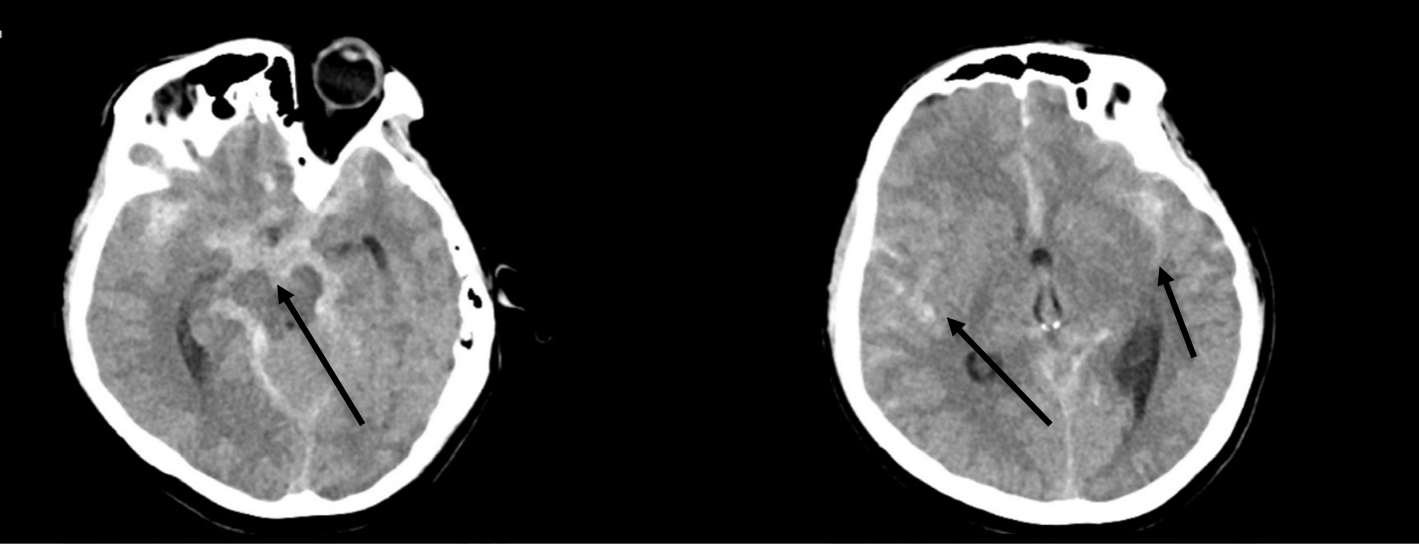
Computed tomography of the head showing large subarachnoid hemorrhage and blood in the fissures (arrows)
